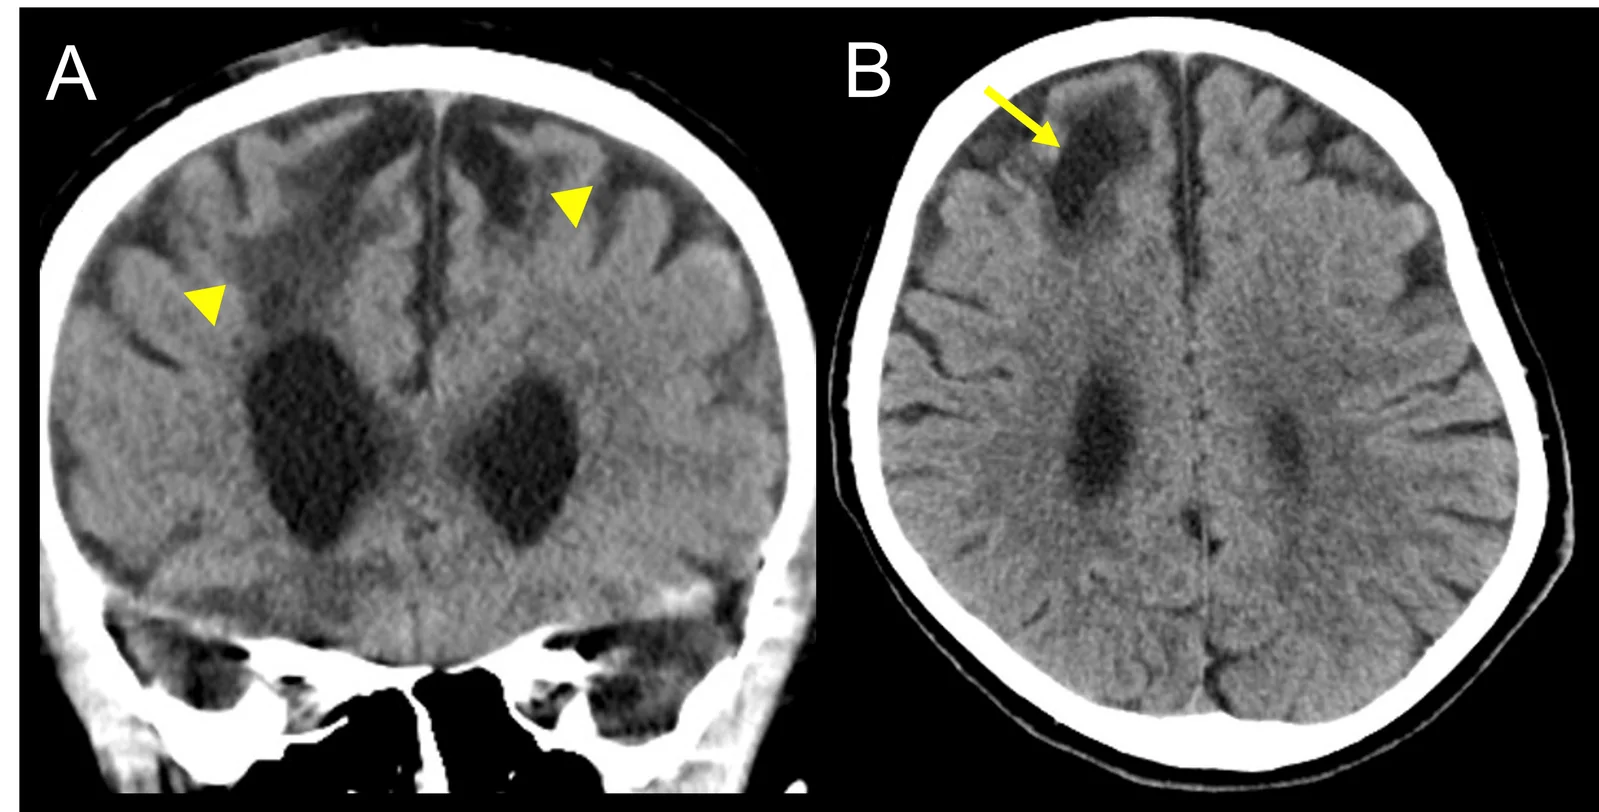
Head CT obtained two years after traumatic brain injury (A) Coronal reconstructed image. (B) Axial image. Low-density lesions involving the cortex and subcortical white matter of the bilateral frontal lobes, predominantly affecting the superior frontal gyri (arrowheads). Cystic changes are observed within the superior frontal lesions, with greater involvement on the right side (arrow) CT: computed tomography
